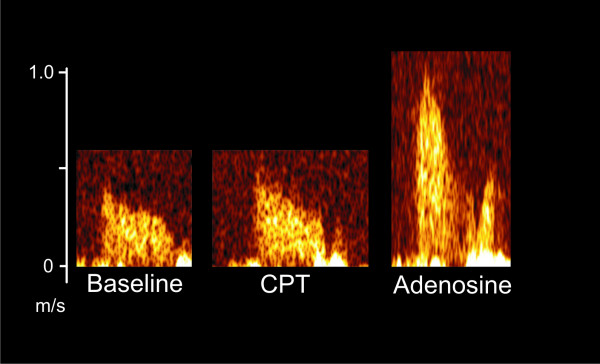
Coronary flow velocity in the LAD obtained using pulsed-wave Doppler at baseline, during cold pressor test (CPT) and adenosine infusion.
